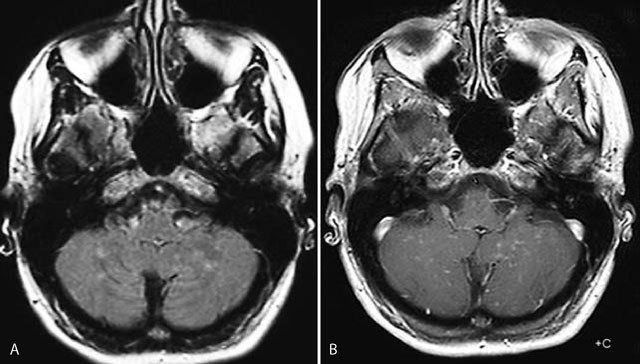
Axial FLAIR image (A): multiple punctate and linear FLAIR hyperintense blurry lesions without mass effect (A) predominatly located in the cerebellar vermis and hemispheres, which become more apperent on contrast-enhanced T1-weighted image (B).
